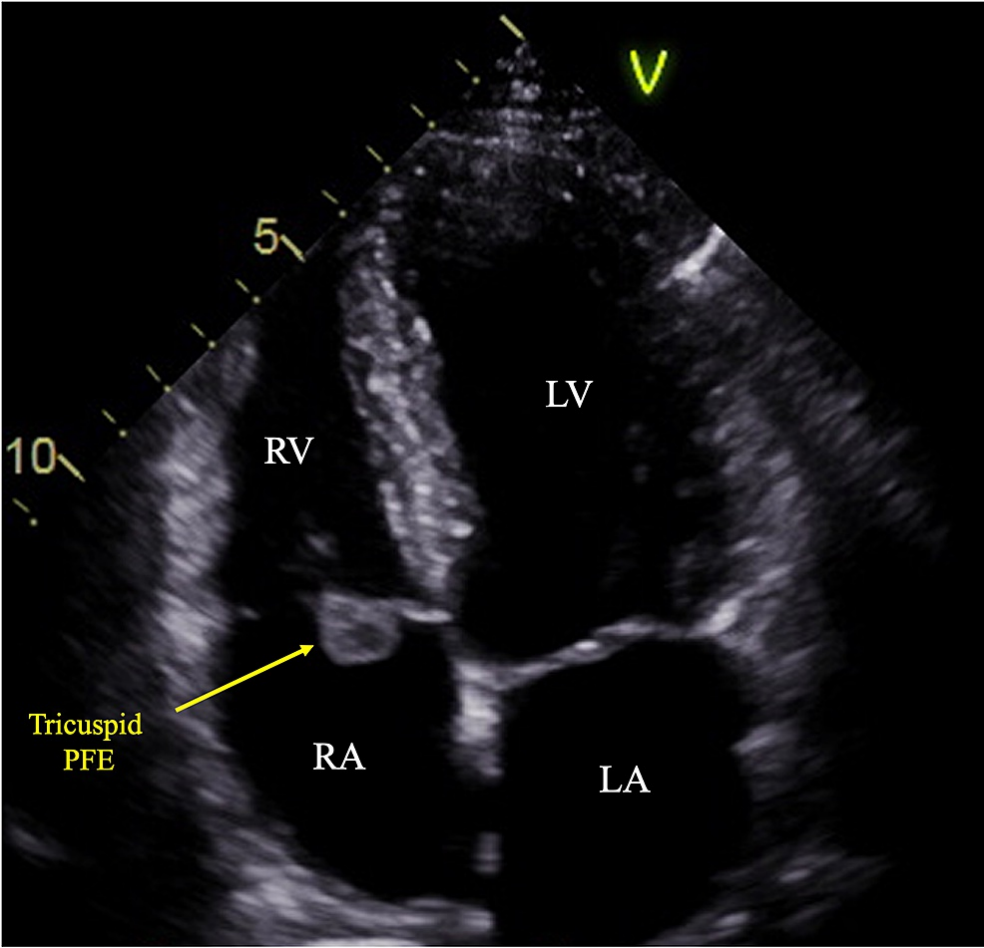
Apical four-chamber view on echocardiography visualizing a mass on the atrial side of the septal leaflet of the tricuspid valve. LA, left atrium; LV, left ventricle; RV, right ventricular; RA, right atrium; PPE, papillary fibroelastoma
